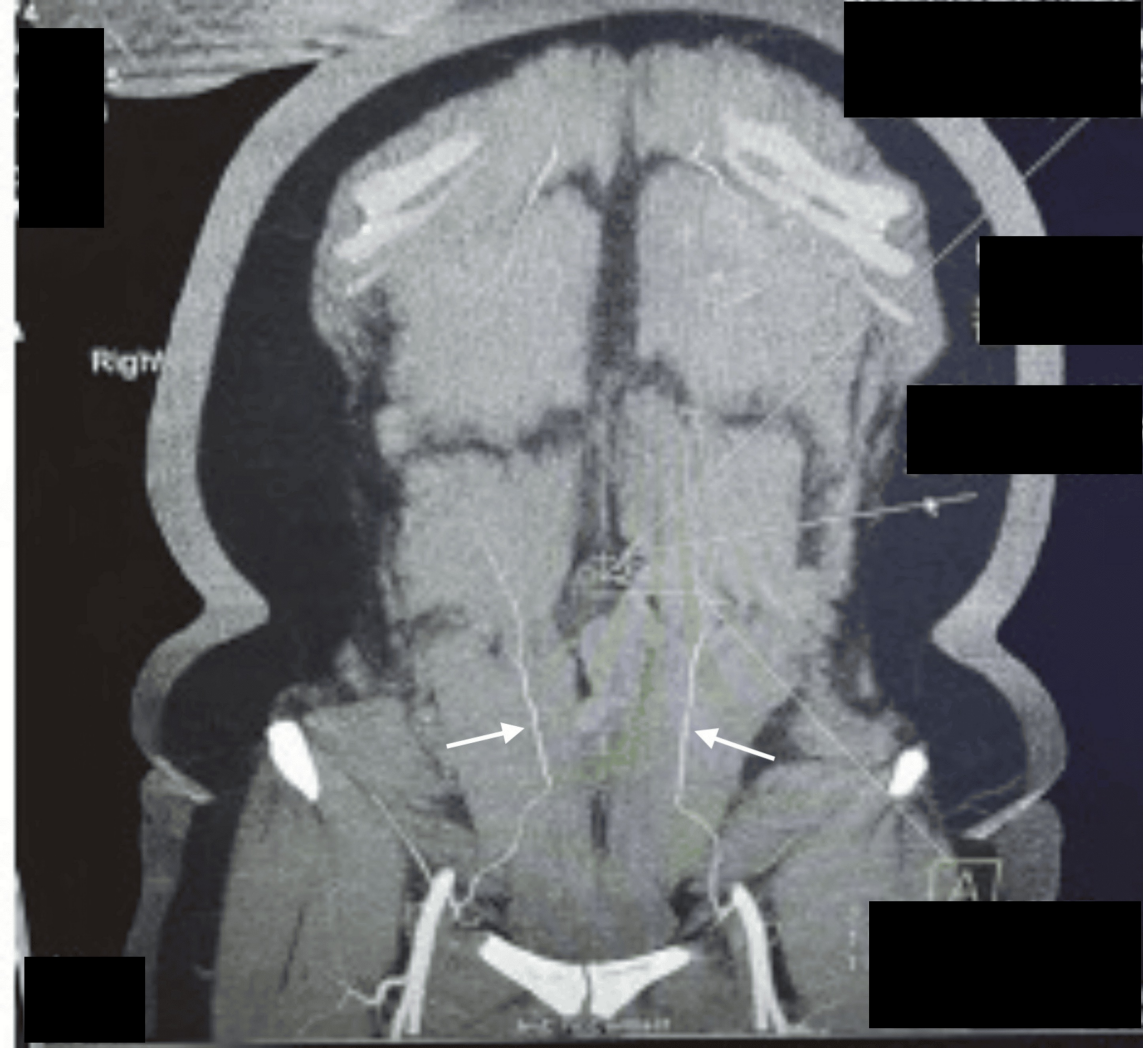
Preoperative CT angiogram (coronal view) showing superficial system dominance
